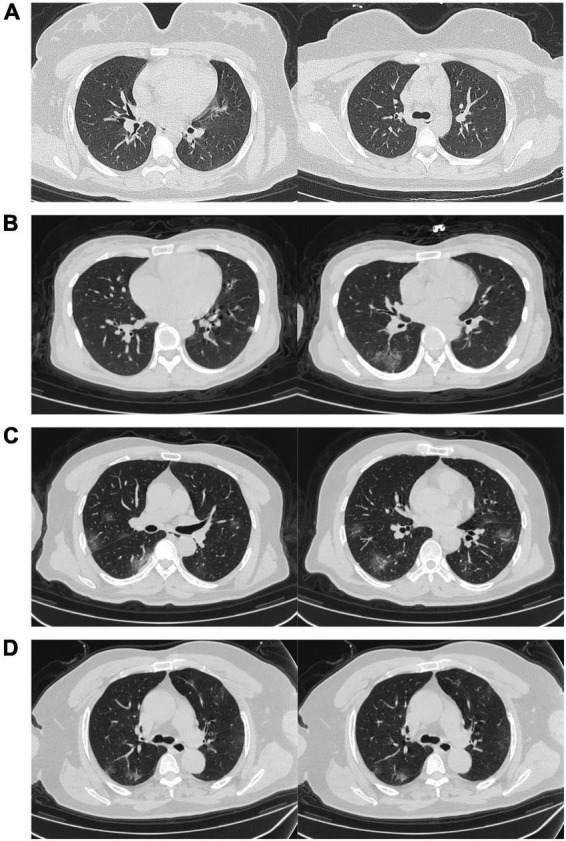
Representative chest CT images. (A) Transverse chest CT images for a 16-year-old female infected with Omicron only showing ground-glass opacity on day four after symptom onset. (B) Transverse chest CT images for a 35-year-old female infected with Omicron showing signs of bilateral pneumonia on day four after symptom onset. (C) Transverse chest CT images for a 51-year-old male infected with Omicron showing signs of bilateral pneumonia on day three after symptom onset. (D) Transverse chest CT images for a 73-year-old male infected with Omicron showing multiple bilateral pneumonia on day six after symptom onset.
